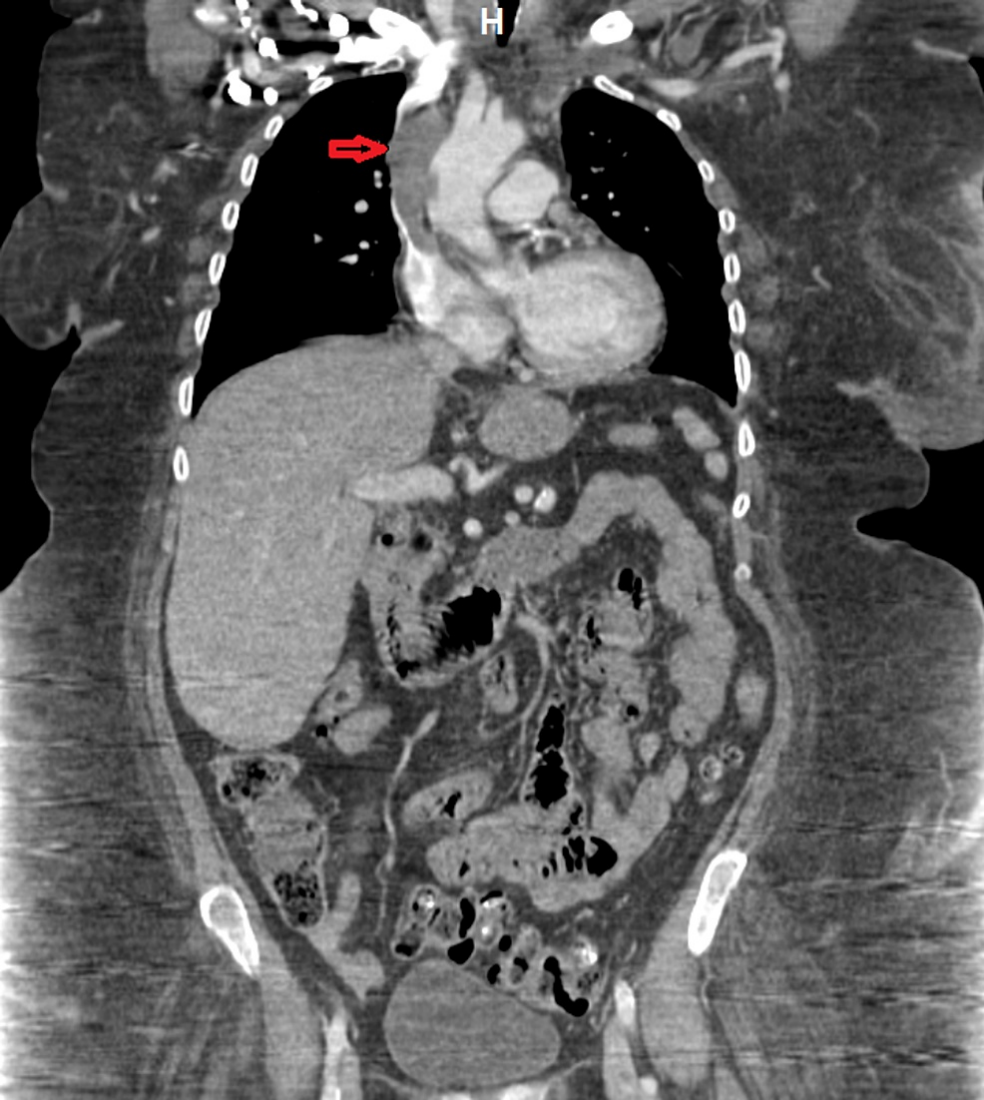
CT of the chest/abdomen/pelvis (coronal view). The red arrow shows soft tissue density in the SVC with contrast flowing around the periphery consistent with acute SVC thrombosis. CT: computed tomography; SVC: superior vena cava
